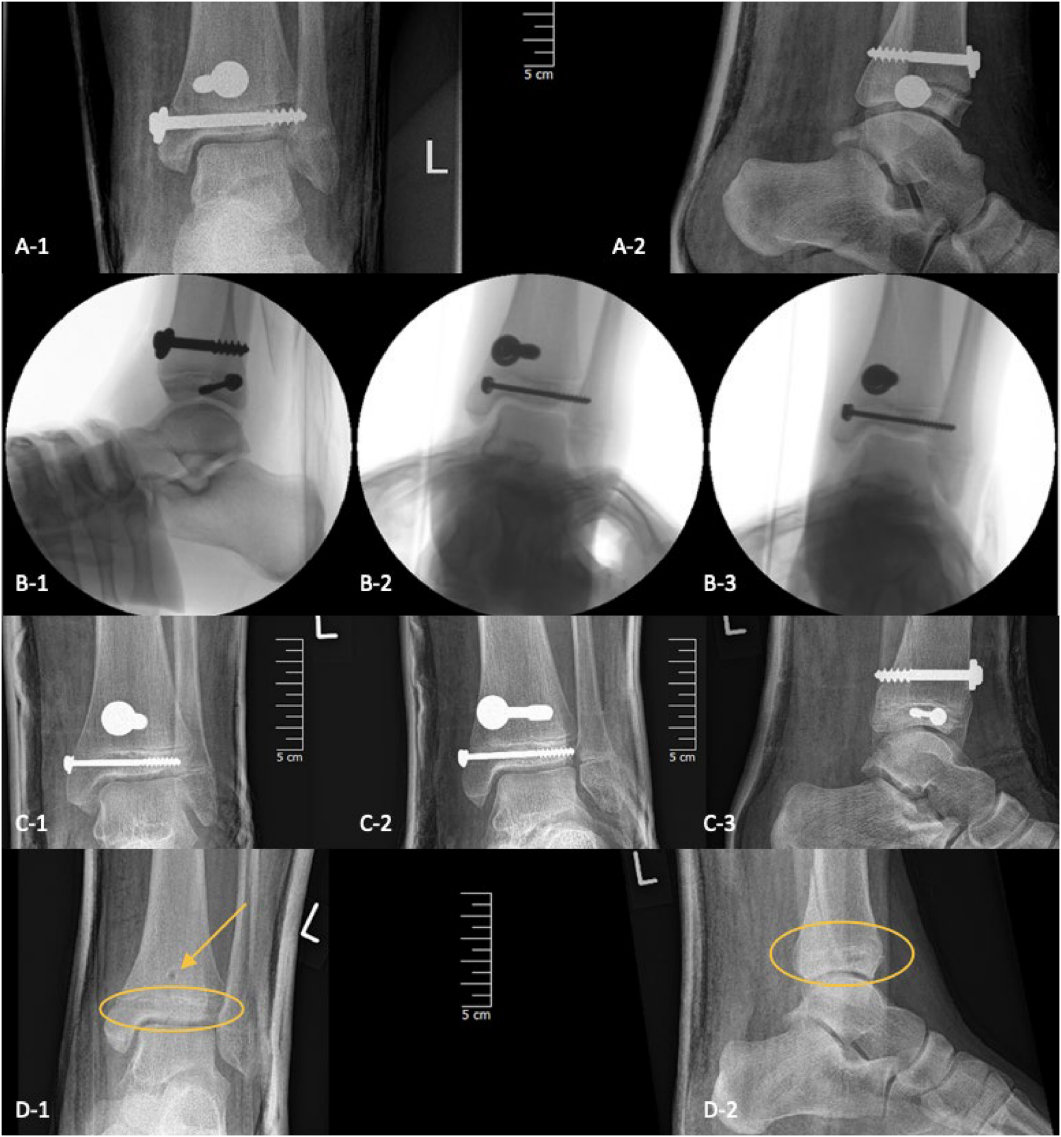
Three-plane radiographs of SH-IV fracture one-day post-op in three children treated with metal (A/B/C-1/2/3) and two-plane radiographs in another treated with PLGA implants (D-1/2), the PLGA implant is marked with the yellow oval and arrow.
